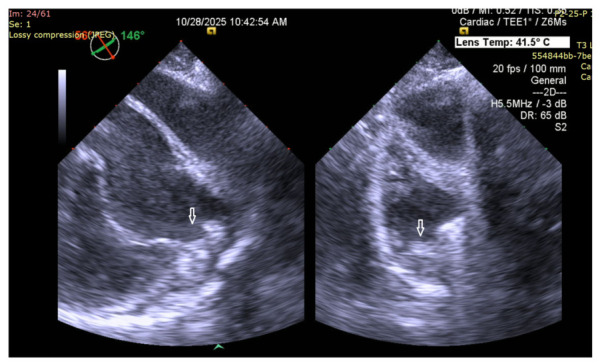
Echocardiographic confirmation of complete LAA inversion. With continued suction, TEE views at approximately 56° and 146° show complete inversion of the LAA into the left atrium (arrows), forming a smooth tissue mound consistent with mechanical inversion from negative pressure.
